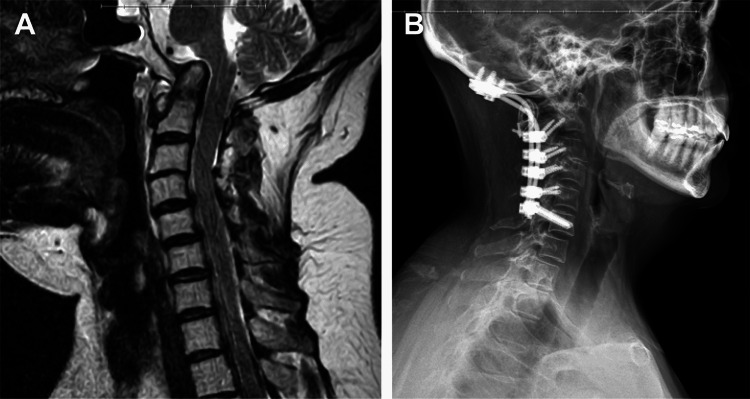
A woman in her early 40s presented with basilar invagination causing cervicomedullary compression in the setting of rheumatoid arthritis. (A) Preoperative sagittal MRI showed cervicomedullary compression. The patient was treated with a fusion from the occiput to C6. Pedicle screws were placed at the distal fixation point at C6 using the described freehand technique with direct visualization. (B) Hardware remained intact on three-year follow-up lateral radiographs. Used with permission from Barrow Neurological Institute, Phoenix, Arizona.
